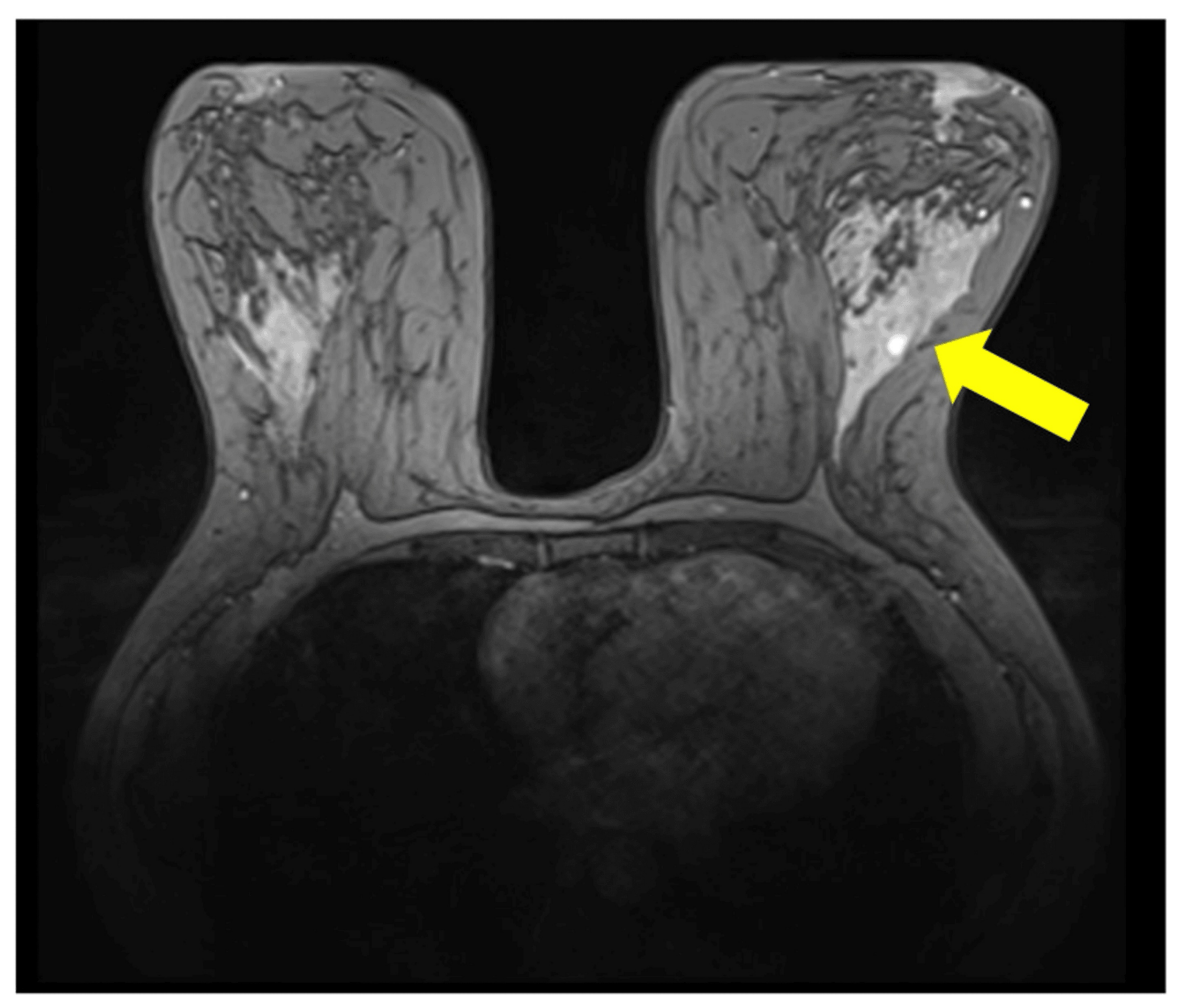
Breast MRI: left breast ectasia, with inflammatory infiltration (mastitis, arrow). MRI, magnetic resonance imaging
